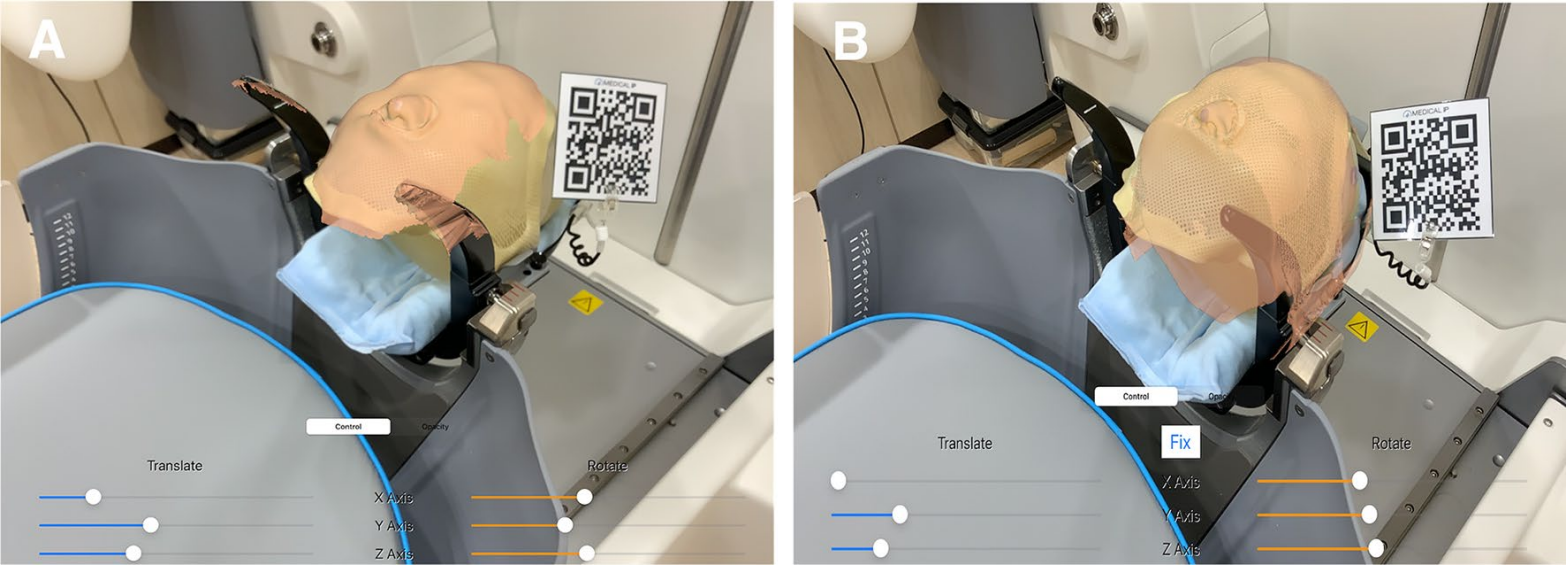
The virtual models and a 3D-printed model overlap correctly using fixed-augmented reality. The virtual model based on 3D-scanning (A) and that based on cone-beam computed tomography (B) are shown.
